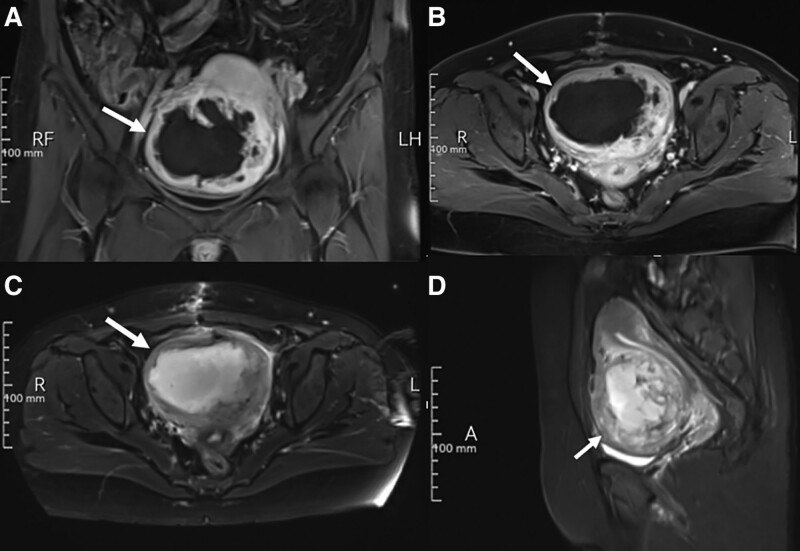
MRI scan revealed an 8-cm in diameter mass with thick wall in the anterior myometrium. The mass was solid-cystic (arrow), with mixed low intensity on T1-weighted (fat suppressed) images (A and B) and mixed high intensity on T2-weighted images (C and D). MRI scan suggested leiomyoma degeneration, along with necrosis and infection of the surrounding tissue. MRI = magnetic resonance imaging.
